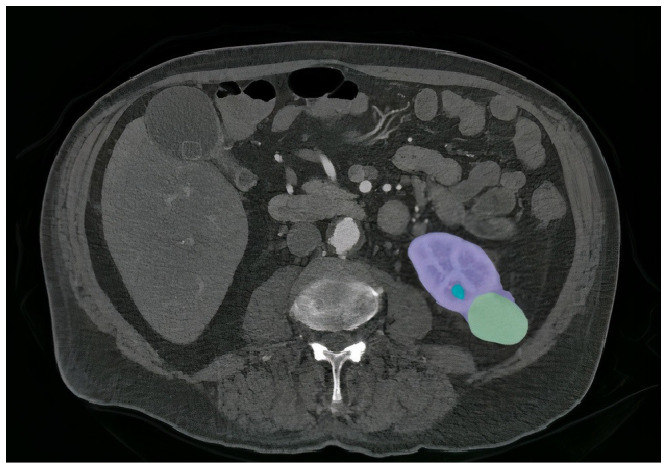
An example of a segmented slice from volume of CT scan Modality. The kidney region is shown in purple, the tumor is shown in green, and the cyst is shown in blue.
